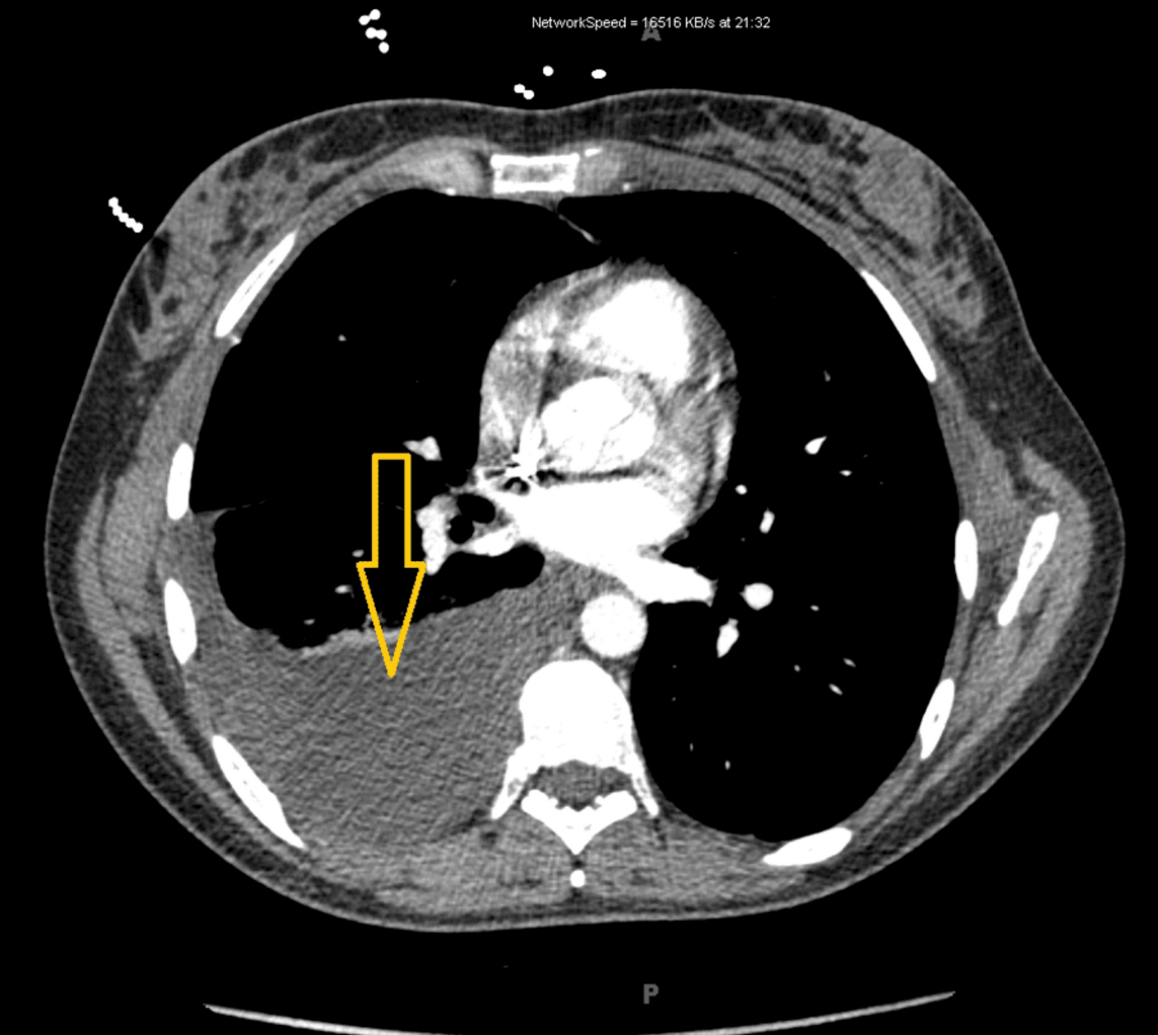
CT scan of the chest This CT scan of the chest shows a large pleural effusion (indicated by the yellow arrow).
